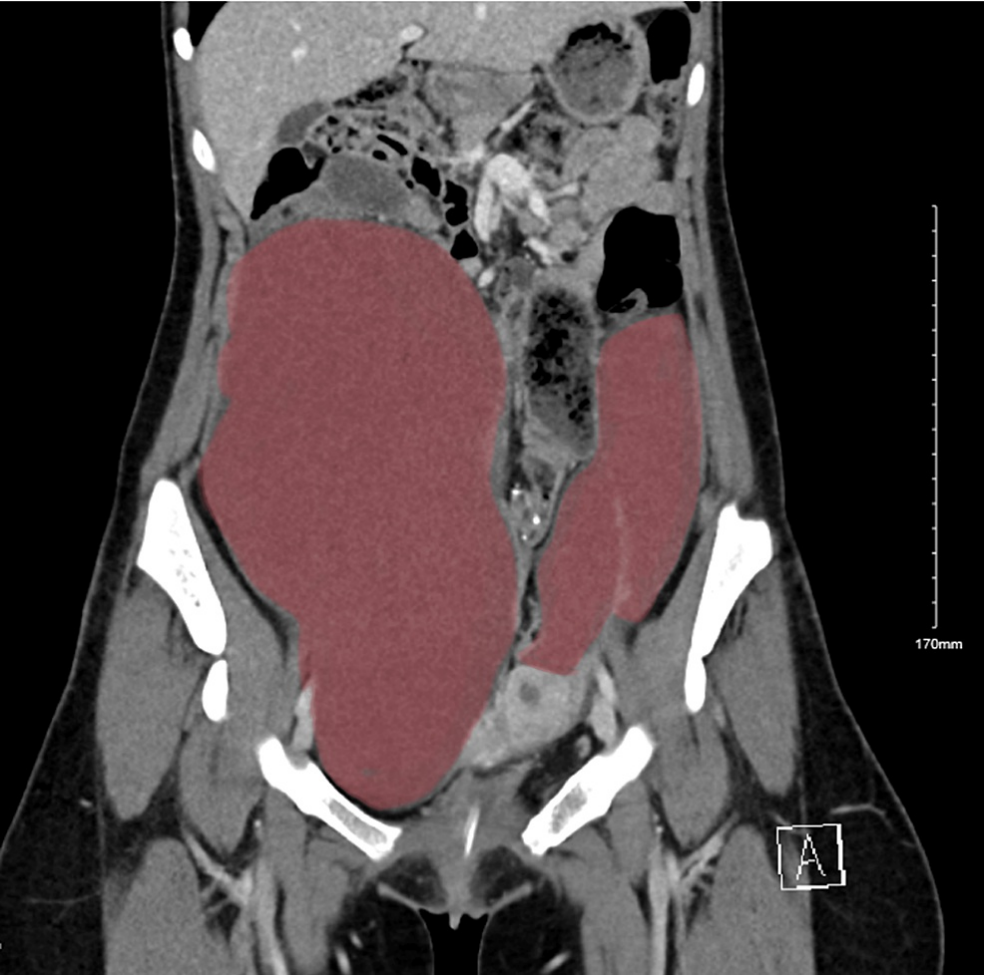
CT scan of the abdomen and pelvis of the bilateral peritoneal cysts in the coronal view.
